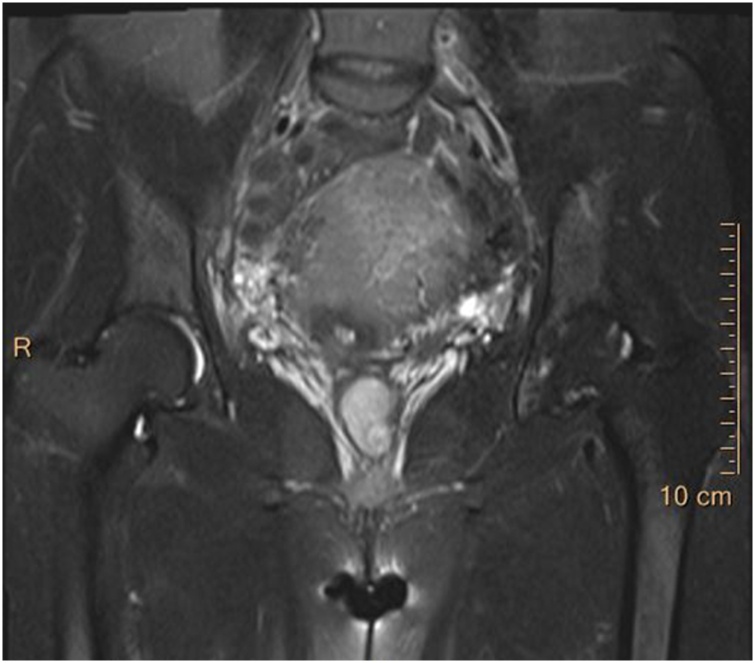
MRI longitudinal section.
